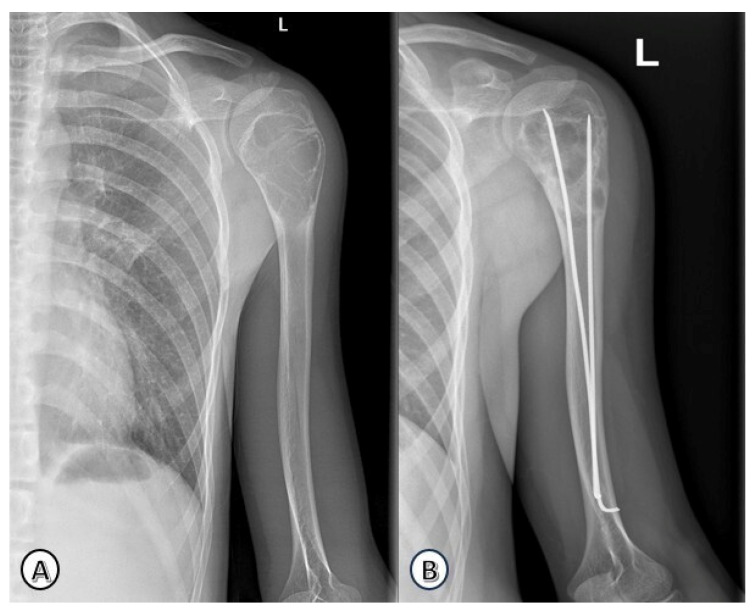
Radiographic evolution of a humeral solitary bone cyst in a 15-year-old boy. (A) Preoperative anteroposterior view of the left proximal humeral metaphysis showing a cystic lesion. (B) Follow-up radiograph at 1-year post-treatment with ESIN, demonstrating gradual remodeling and cortical thickening.
